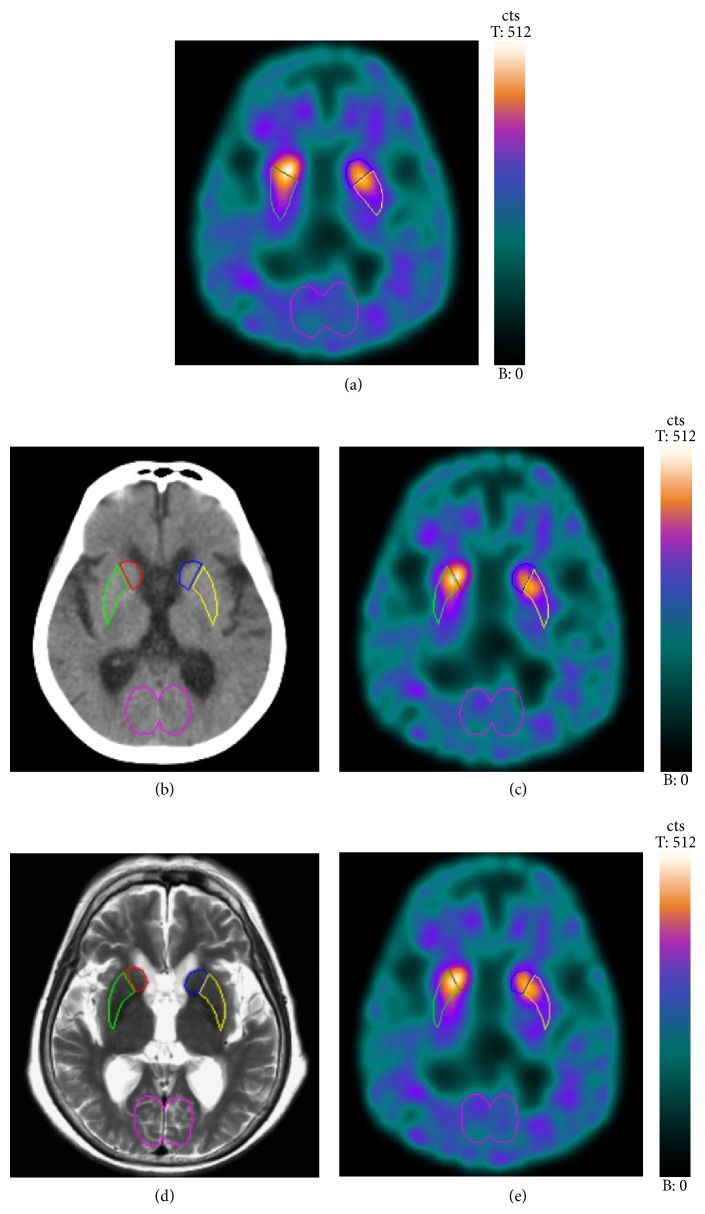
Regions of interest (ROIs) were manually drawn directly on the summed SPECT image (a). In CT-guided method, ROIs were manually delineated directly on one CT slice with the best recognizable striatum. ROIs were then transferred to the hardware-based coregistered summed SPECT image (b, c). In MRI-guided method, ROIs were manually delineated directly on one MRI slice with the best recognizable striatum. ROIs were then transferred to the software-based coregistered summed SPECT image (d, e).
